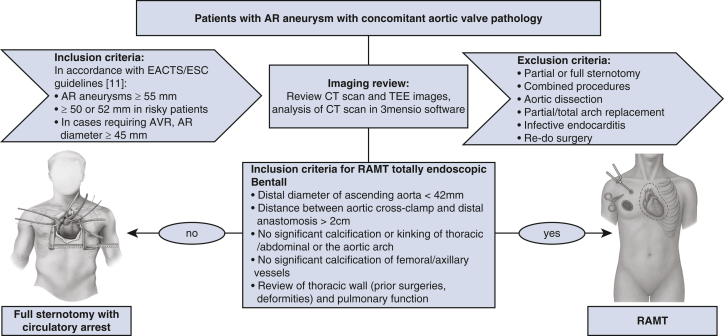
Patient selection and screening algorithm for totally endoscopic Bentall procedure via RAMT. *RAMT*, Right anterior minithroacotomy; *AR*, aortic root; *EACTS*, European Association for Cardio Thoracic-Surgery; *STS*, Society of Thoracic Surgeons; *AVR*, aortic valve replacement; *CT*, computed tomography; *TEE*, transesophageal echocardiography.
